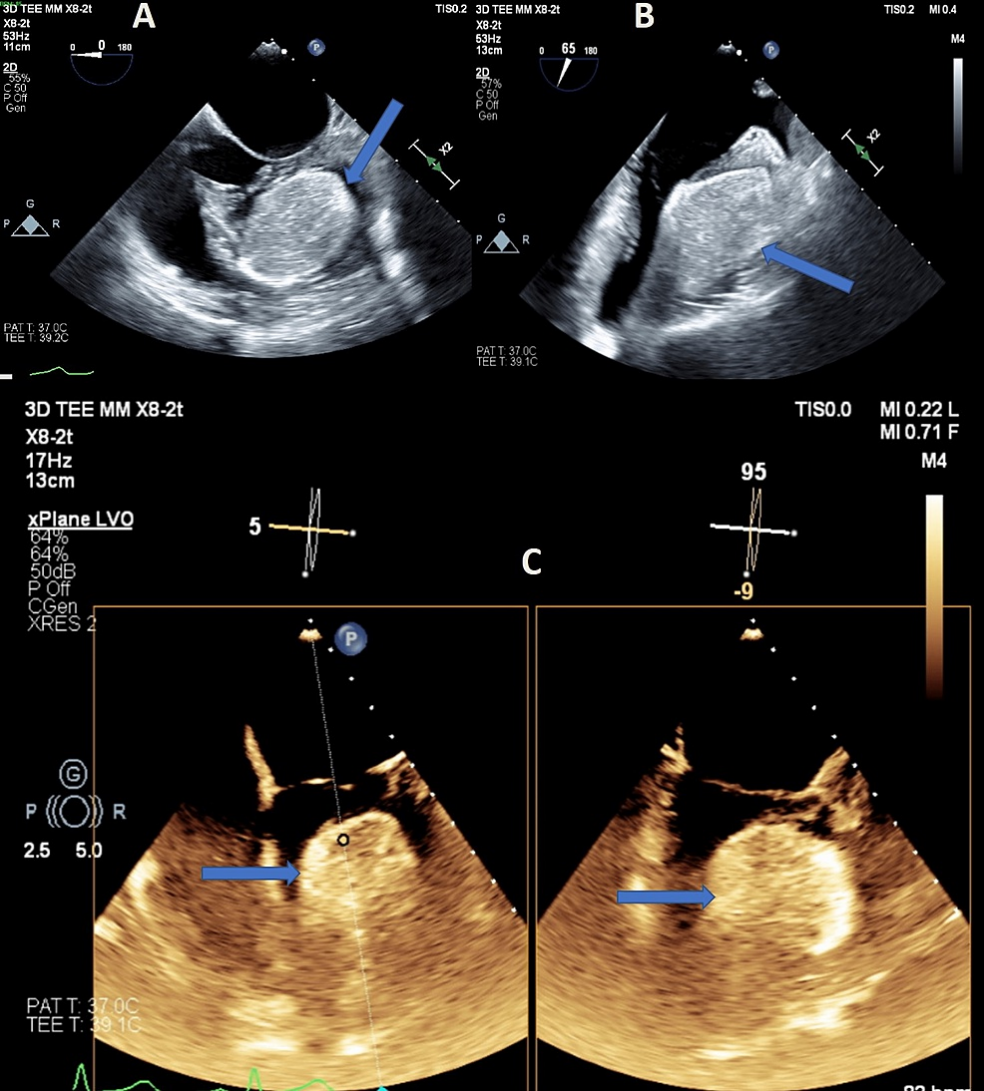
Transesophageal echocardiogram Four-chamber (A) and two-chamber (B) views. (C) Contrast-enhanced four-chamber view with X-plane imaging showing left-ventricular lipoma (blue arrows).
